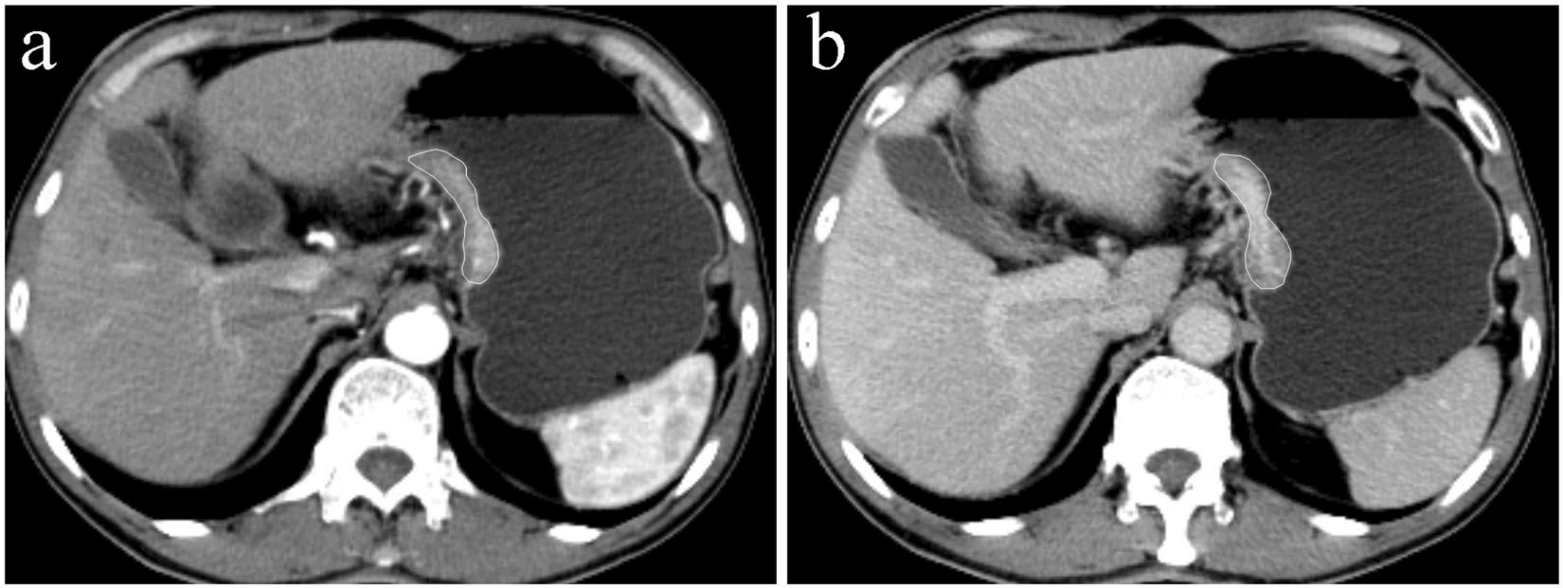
A 66-year-old man with poorly differentiated gastric adenocarcinoma positive for E-cadherin expression (+++), negative for Ki67 expression (expression index: 50%), weakly positive for VEGFR2 expression and weakly positive for EGFR expression. Axial CT images in the (a) arterial and (b) venous phases show a thickened wall with remarkable enhancement in the lesser curvature of the stomach. Note the region of interest (ROI) covering the largest slice of the lesion.
